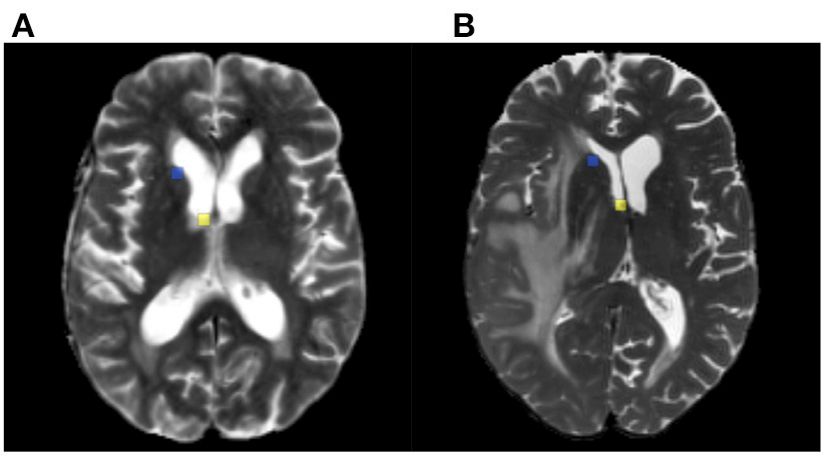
Example of annotated landmarks on test set. Panels (A,B) correspond to post and pre-operative MRI acquisitions of the same patient. The corresponding landmarks are visualized with the same colors.
